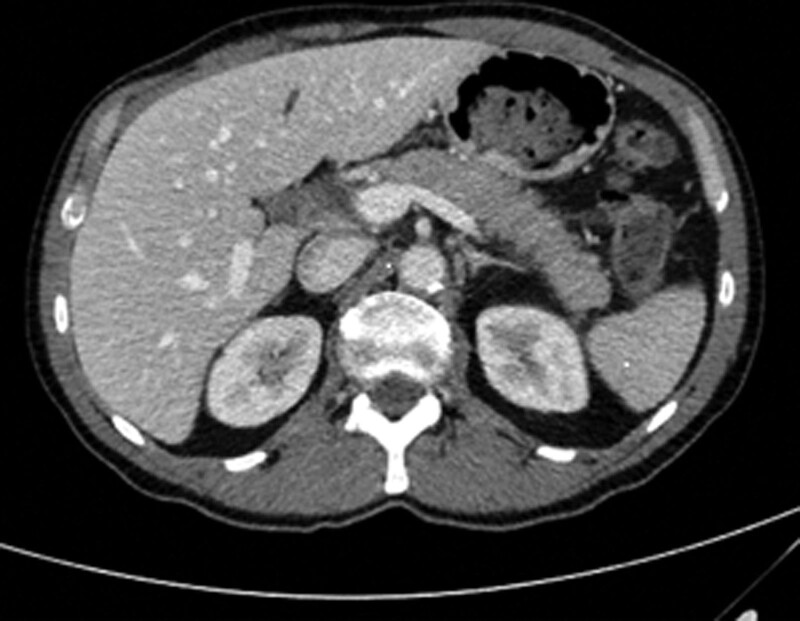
Abdominal CT scan showing swollen pancreas. CT = computed tomography.
